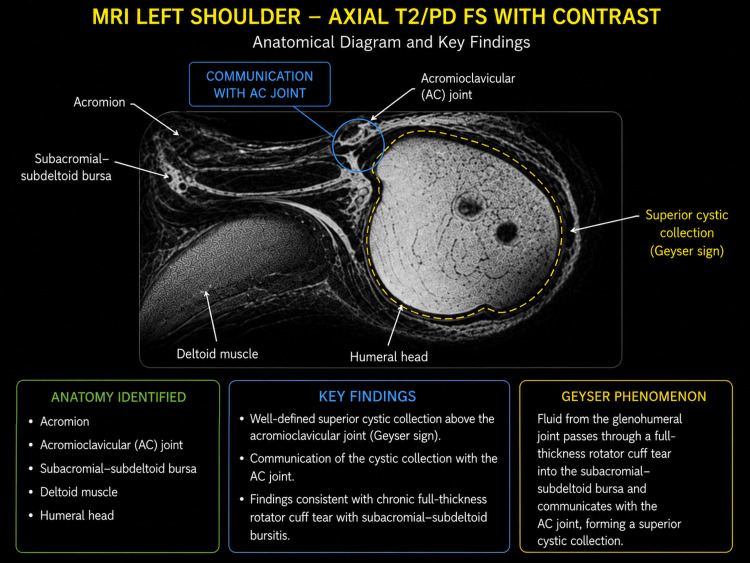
Axial MRI image of the current patient, demonstrating a cystic lesion communicating with the acromioclavicular (AC) joint, consistent with the Geyser phenomenon. Arrows indicate the communication pathway between the cyst and the acromioclavicular joint. Note: Annotations were added by the authors using Canva® (Canva Pty Ltd., Sydney, Australia) based on the patient’s original MRI image. No AI-generated images were used.
